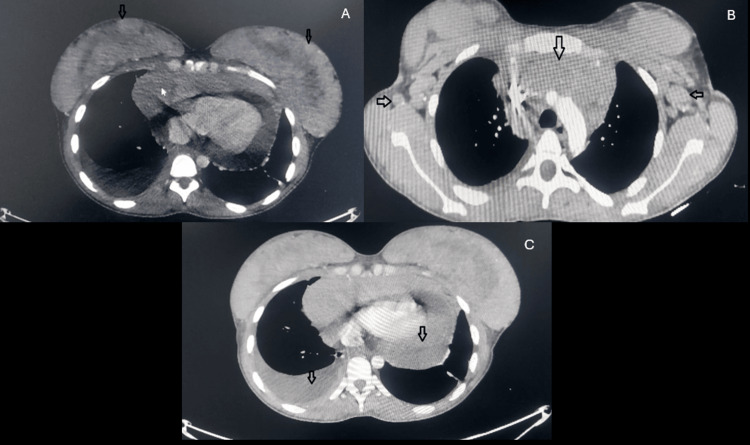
CT scan showing bilateral breast enlargement (A), mediastinal mass and bilateral axillary LNE (B), and pleural and pericardial effusion (C). CT: computed tomography; LNE: lymph node enlargement.
